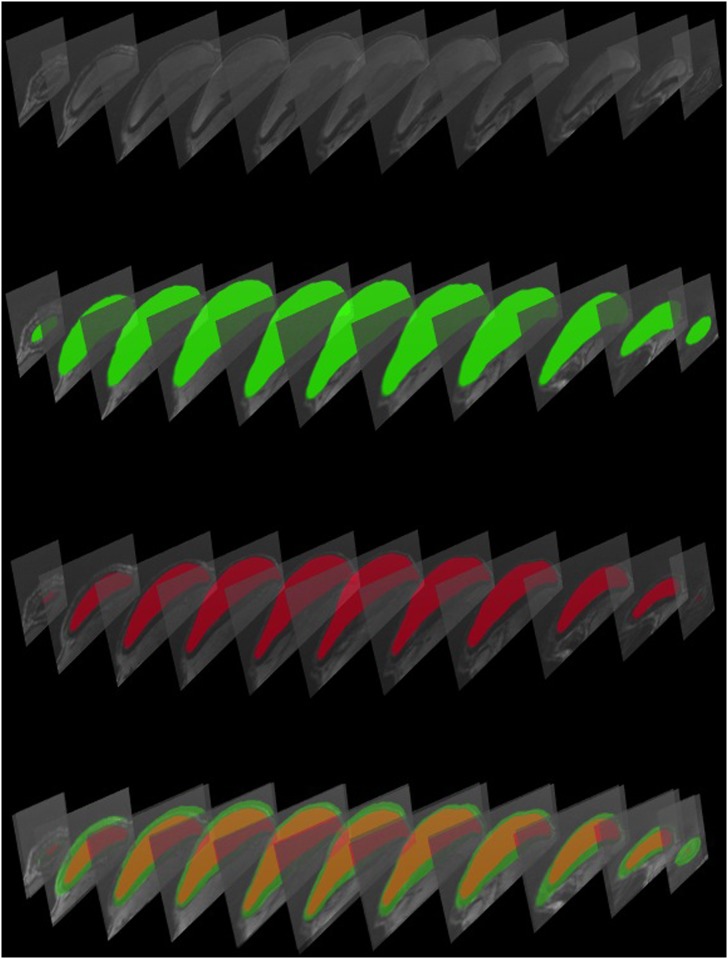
Example pictures of MR images for volumetric measurements. From top to bottom: Blank slides, complete capsule volume (green), implant volume (red) and complete minus implant volume (green margin). Volume calculations were performed via VOLUMEST and the scale was adjusted automatically by the software.
